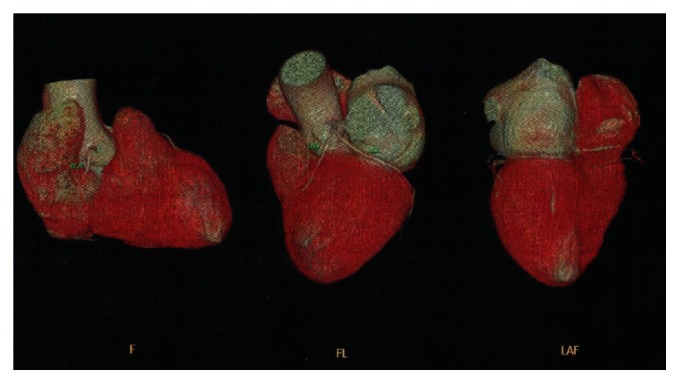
CT coronary angiography. There is no significant stenosis in the coronary arteries.
